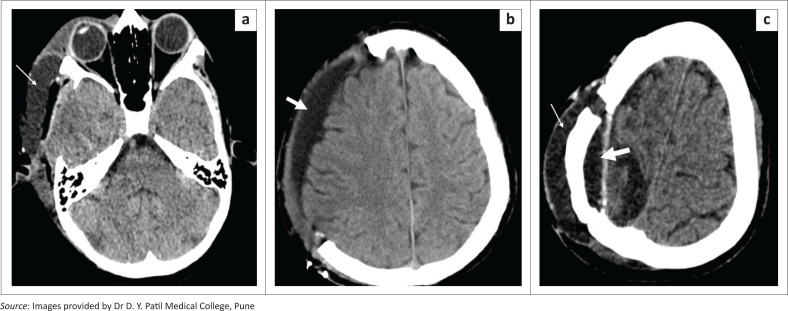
Axial soft tissue window CT image (a) in a patient with a right temporal decompressive craniectomy reveals a subgaleal hypodense fluid collection in the right temporal region (white arrow), suggestive of a subgaleal hygroma. Axial soft tissue window CT image (b) in another patient with a right fronto-parietal decompressive craniectomy demonstrates an extra-axial hypodense fluid collection in the subdural region along the cerebral convexity (white arrow), consistent with subdural hygroma. Axial soft tissue window CT image (c) with a right parietal craniotomy indicating subdural (thick white arrow) and subgaleal (thin white arrow) hygromas.
